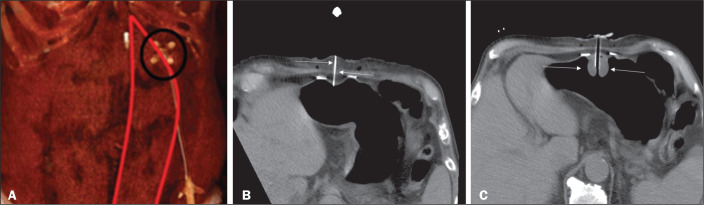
CT-guided retrograde gastrostomy. In A, gastropexy. In B, puncture
